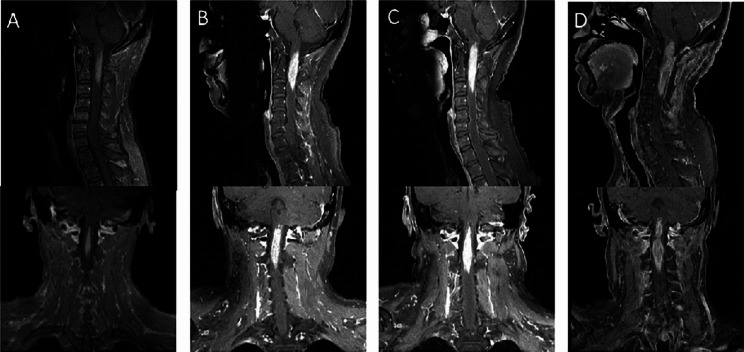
Serial coronal and sagittal postcontrast MR images at presentation and follow-up. Coronal and sagittal contrast-enhanced MRI (A) revealed patchy enhancement in the medulla oblongata and cervical spinal cord at presentation. Repeated enhanced-MRI after 1.5 months (B) showed an enlarged enhanced lesion. MRI at 2 months from presentation (C) showed a new enlargement of enhanced lesion. MRI at 3 months from presentation (D) revealed a larger lesion
